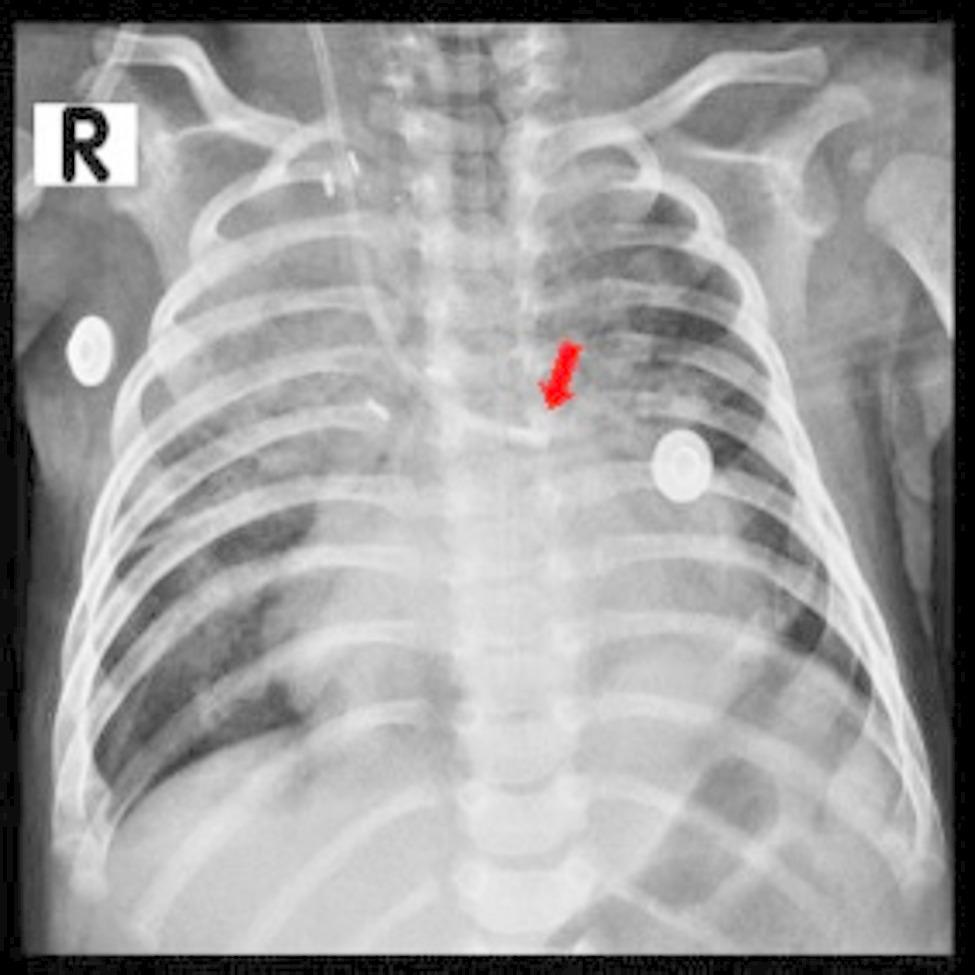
Chest x-ray performed 2 days after central venous catheter (CVC) insertion showing a left-side deviation of the inserted catheter tip (arrow). R, right
